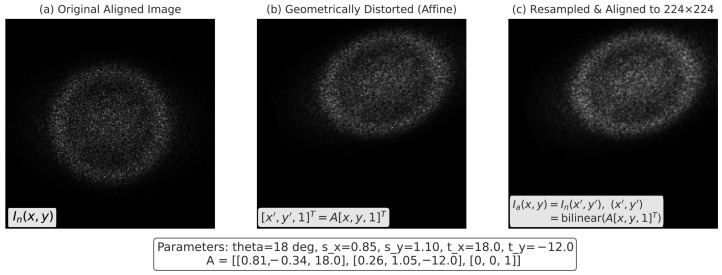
Affine transformation applied to standardize fetal ultrasound views across subjects. This normalization step reduces variability in orientation and scale, facilitating consistent patch embedding and downstream feature extraction.
